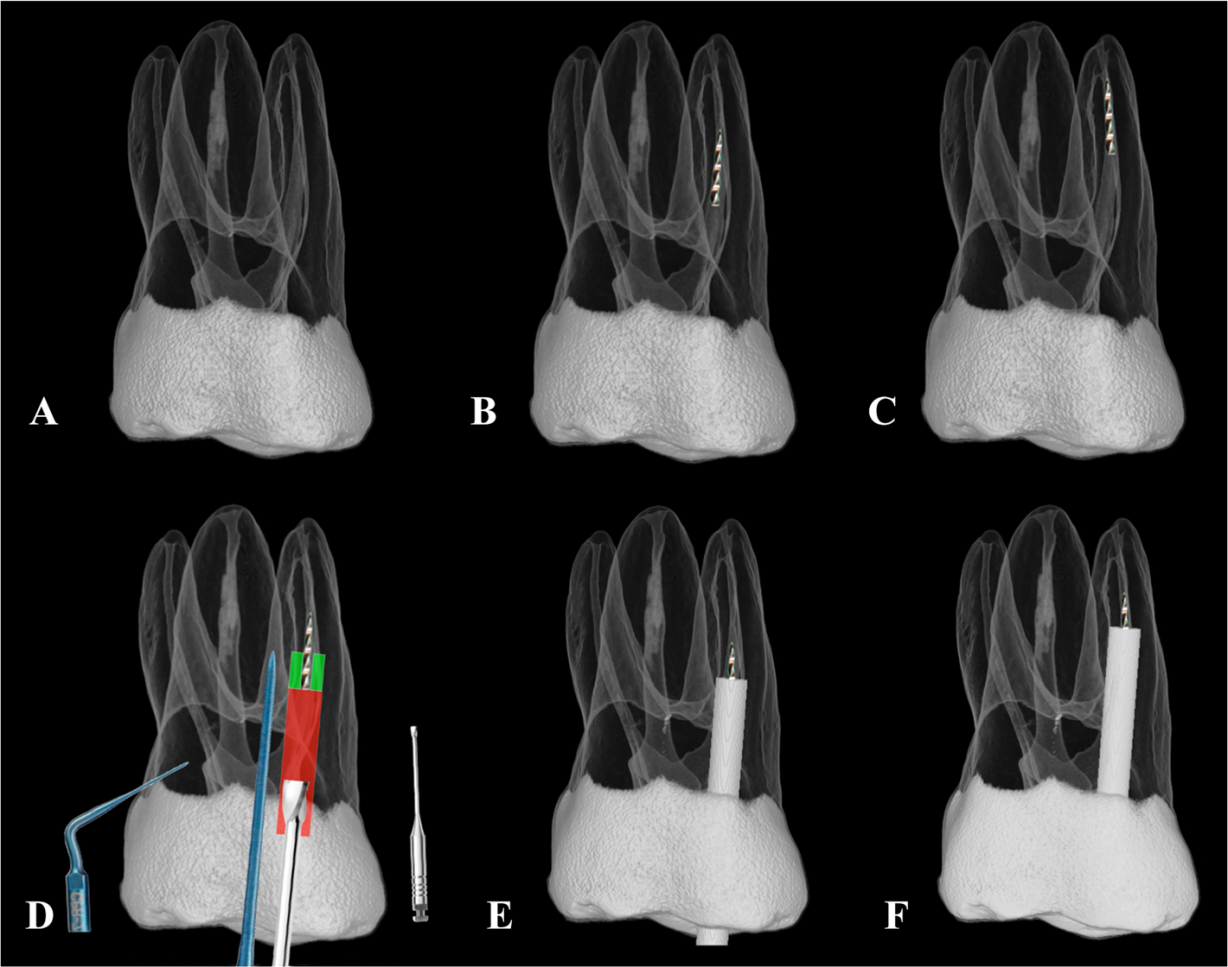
a Morphological reconstruction of one maxillary first molar; b & c size 25/.06 NiTi instrument with 3 mm apical segment assumed to be fractured in the mesiobuccal canal with 3 mm and 5 mm away from the canal orifice; d using modified Gates Glidden burs to create a staging platform and CPR ultrasonic tip to trephine dentin a 1.5 mm distance apically from the coronal part of the fragment around the fragment; e access to the fragment at 3 mm; f access to the fragment at 5 mm
